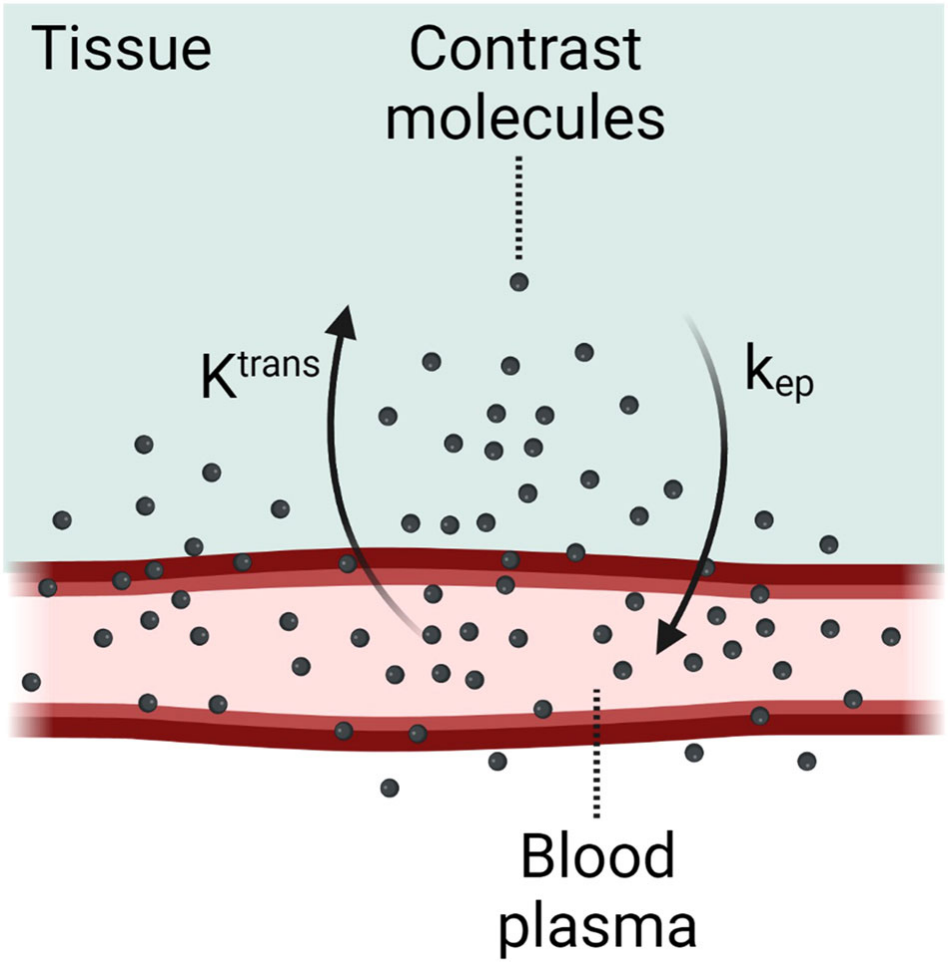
Tofts analysis of dynamic contrast-enhanced MRI (DCE-MRI) allows quantification of contrast agent transfer between the intracapillary space and interstitium. This includes the transfer rate from the capillary into the interstitium (K^trans^) and vice versa (k_ep_). Mathematical modeling (such as Tofts analysis) of DCE-MRI data can be useful when studying the vascular permeability within tissue
